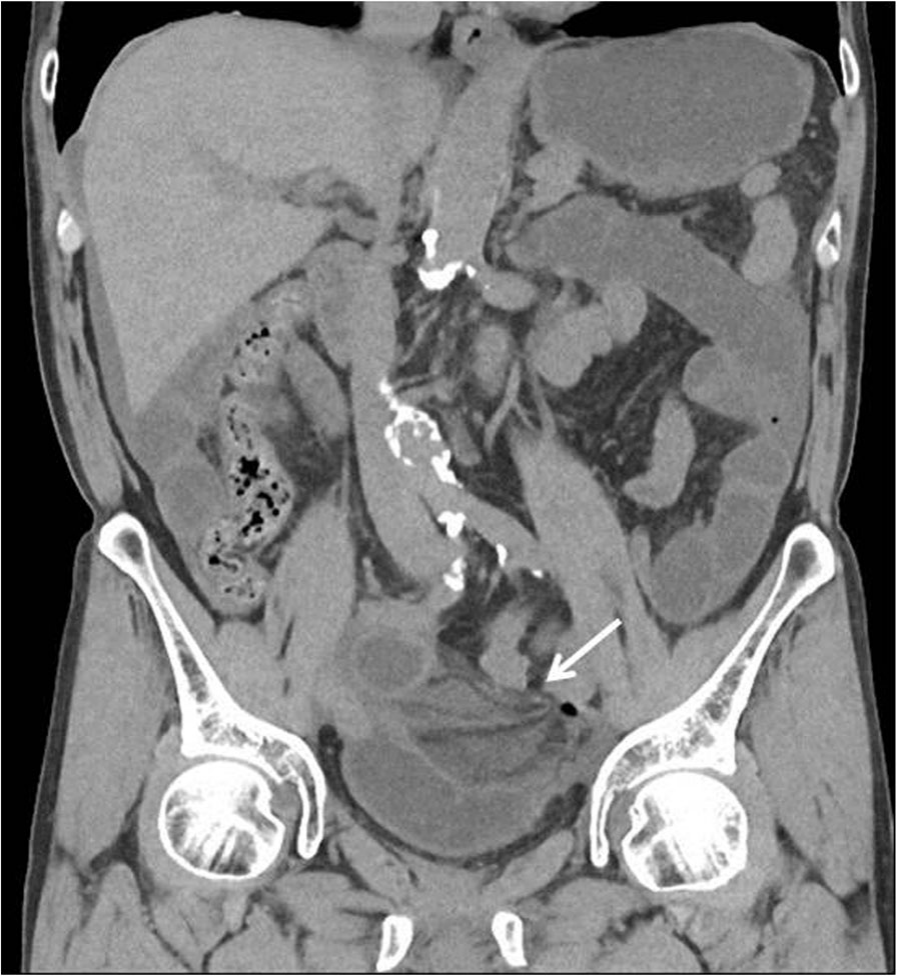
Coronal image of non-contrast computed tomography showed thickened loops of small bowel with surrounding mesenteric edema (arrow) in the left lower quadrant
